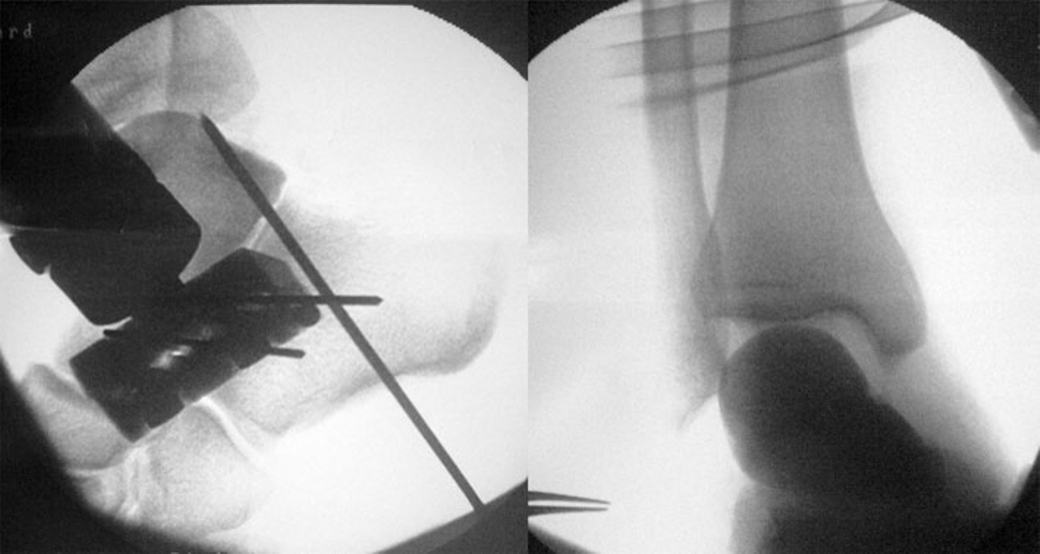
Intraoperative X-rays after application of antibiotic cement
